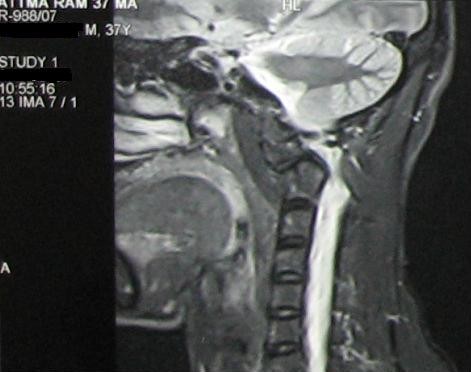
MRI showing cord compression due to anterior translation of the axis over atlas in a subject with atlantoaxial instability.
